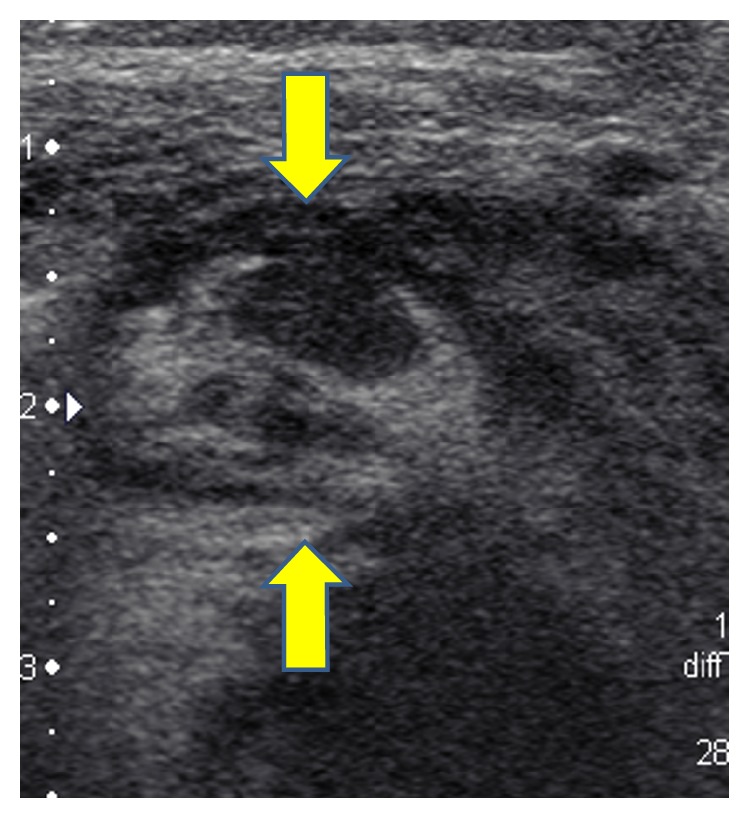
Selected gray-scale axial ultrasound imaging of the right forearm soft tissue mass. A heterogeneous echogenic mass is demonstrated along the ventral forearm musculature displacing, rather than invading, surrounding structures (arrows).
